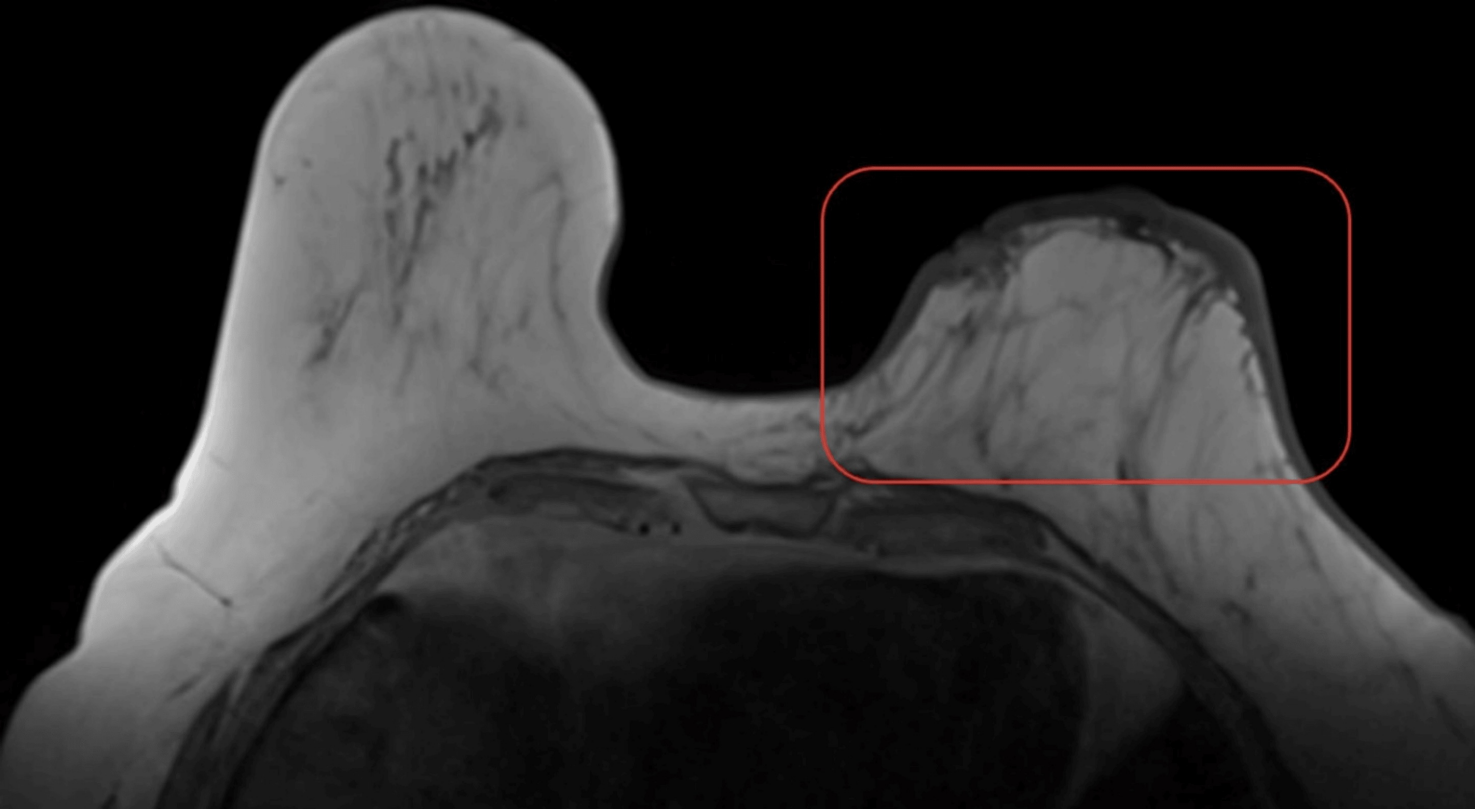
Magnetic resonance imaging (T1-weighted phase, axial view) revealed diffuse skin thickening of the left breast (red box) with increased thickness and nodularity around the nipple area with abnormal parenchymal enhancement just beneath the skin surface.
